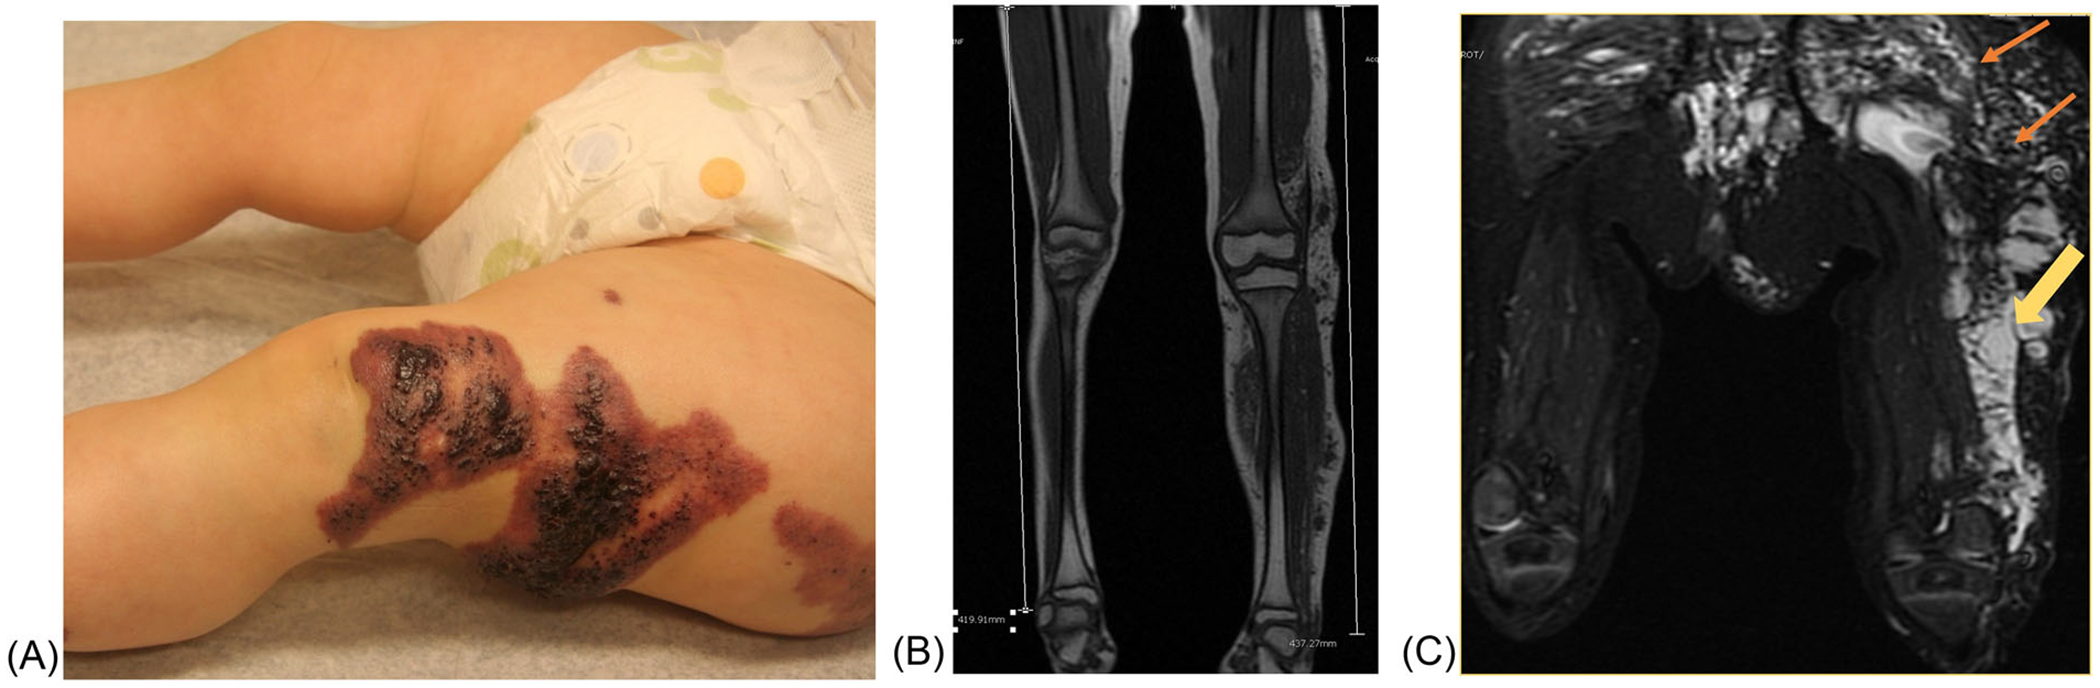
Klippel–Trenaunay syndrome (KTS). (A) KTS or capillary lymphatic venous malformation of the left lower extremity. This disorder is now classified under the genotypic-based term of PIK3CA-related overgrowth syndrome (PROS). Diffuse lymphatic blebs extend over the patient’s leg and perirectal area. These areas are prone to bleeding, leaking, and infection. (B) Limb length discrepancy and subcutaneous changes along the lateral margin of the left lower extremity are noted on T1-weighted magnetic resonance imaging (MRI). (C) Diffusely abnormal soft tissue thickening in the left upper lateral and gluteal compartment demonstrating lymphatic malformation (orange arrows). There are also multiple areas of phlebectasia seen along the lateral compartment of the thigh (yellow arrow)
